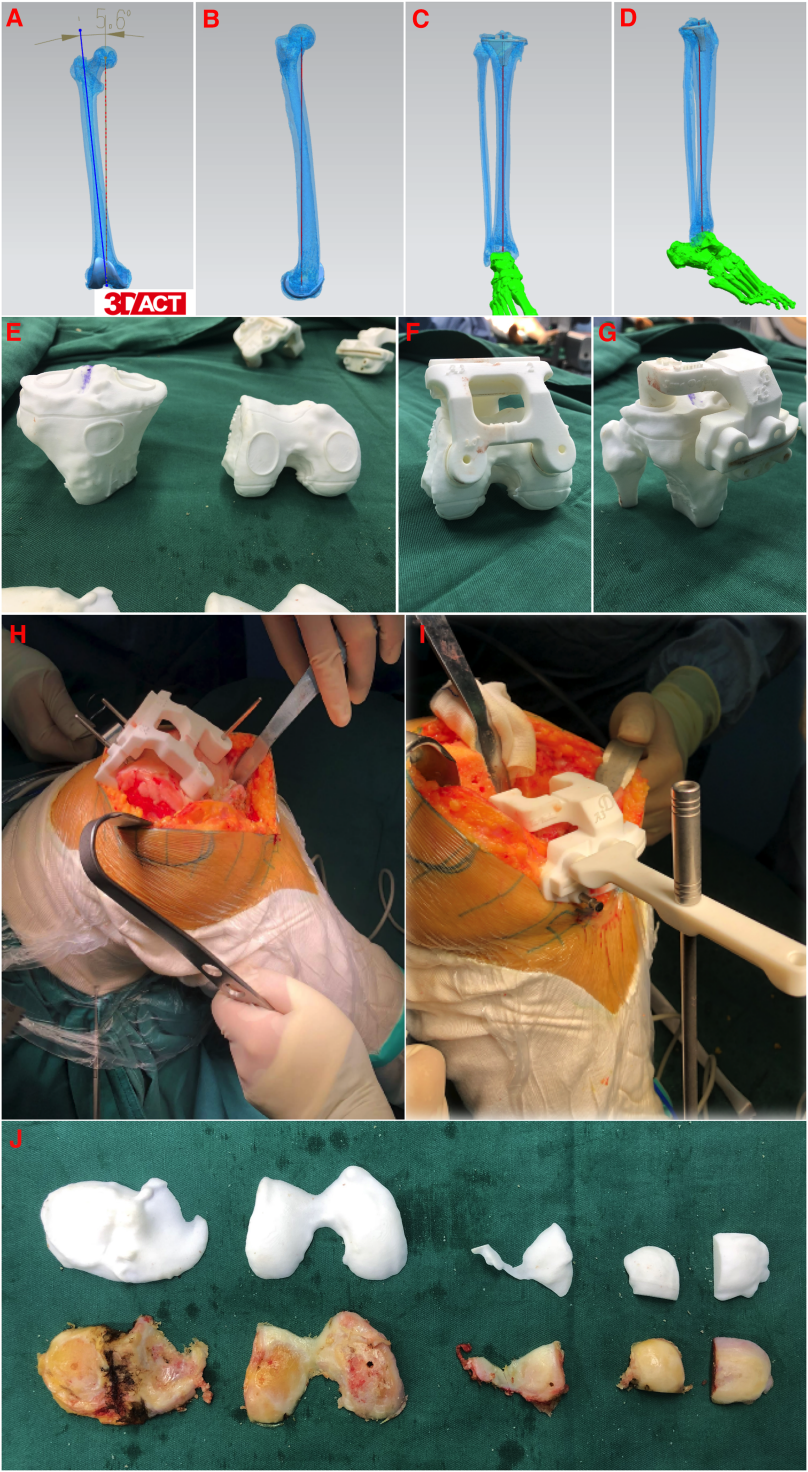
Design and intraoperative application of 3D printed patient-specific instrumentations. (A–D) CT-based preoperative planning of femoral and tibial prosthetic implant for anatomic reconstruction. (E) Reconstructed models of the patient's distal femur and proximal tibia, including markers for the location of the intraoperative osteotomy. (F,G) Confirming the good fit of 3D printed patient-specific instrumentations. (H,I) Intraoperative positioning and application of 3D printed patient-specific instrumentations. (J) Real-time comparison of preoperative designed osteotomy volume and actual osteotomy volume.
